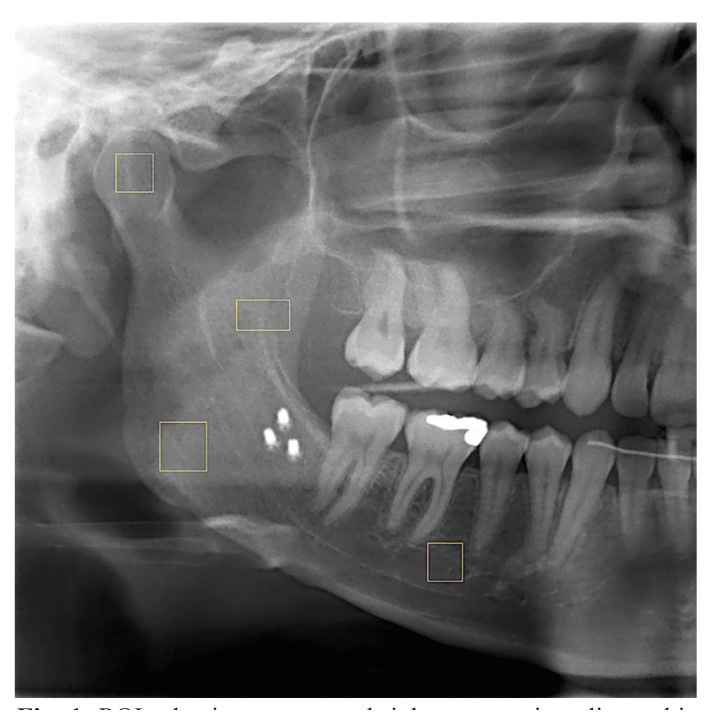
ROI selection on cropped right panoramic radiographic images.
